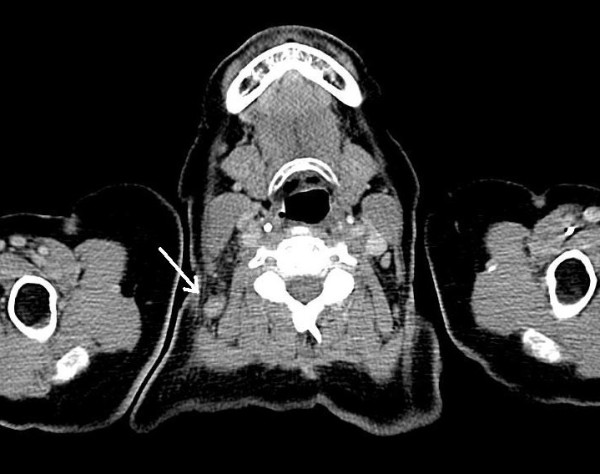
Neck computed tomography scan. Non-necrotizing lymphadenitis (arrow) along the draining lymph nodes without evidence of cervical or mediastinal abscess formation (courtesy of Mathias Langer, University Hospital Freiburg, Germany).
